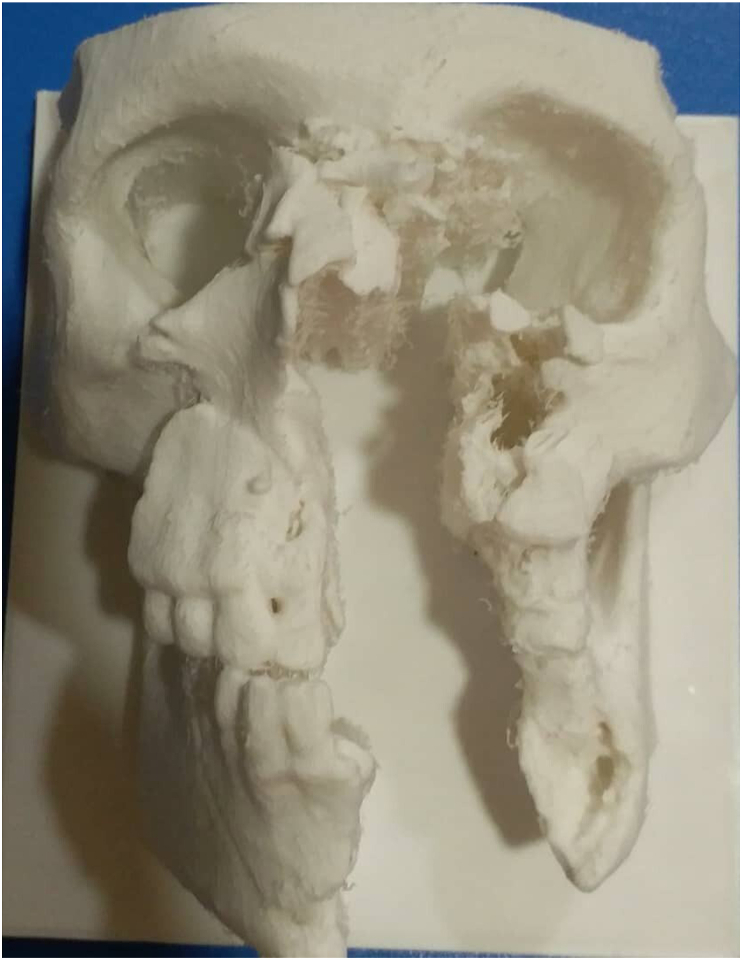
3D reconstruction based on CT scan slices in the first case.
